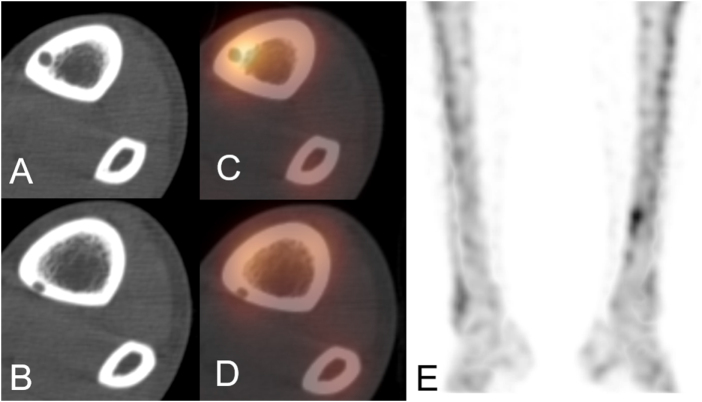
CT images (A and B), CT/SPECT images (C and D), and bone scintigraphy images (E) demonstrating intracortical lesions and abnormal uptake.
